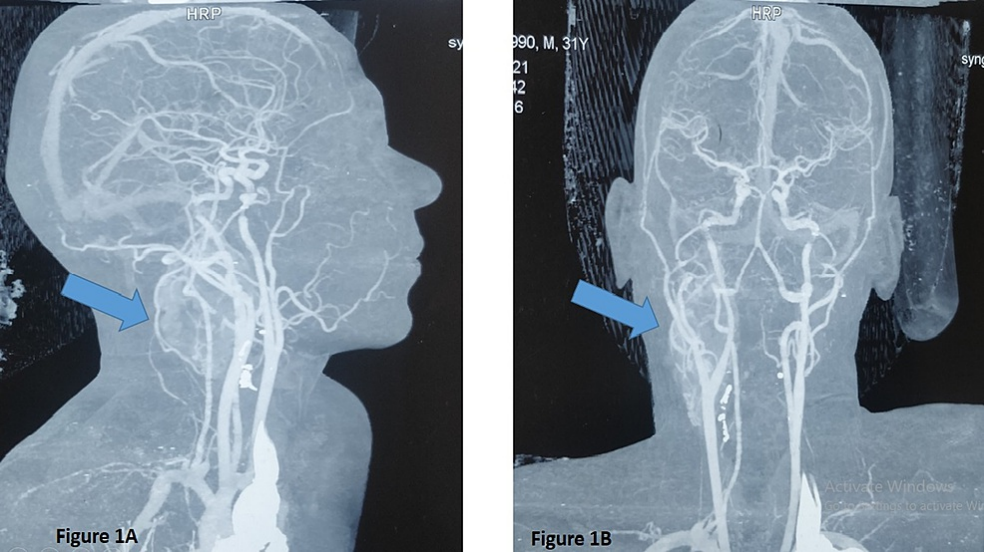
MRI with contrast sagittal view (Figure 1A) and craniocaudal view (Figure 1B) Blue arrow indicates a mass of 50 x 26 x 28 mm on the right side of the neck causing splaying of the internal carotid artery and external carotid artery MRI: magnetic resonance imaging
